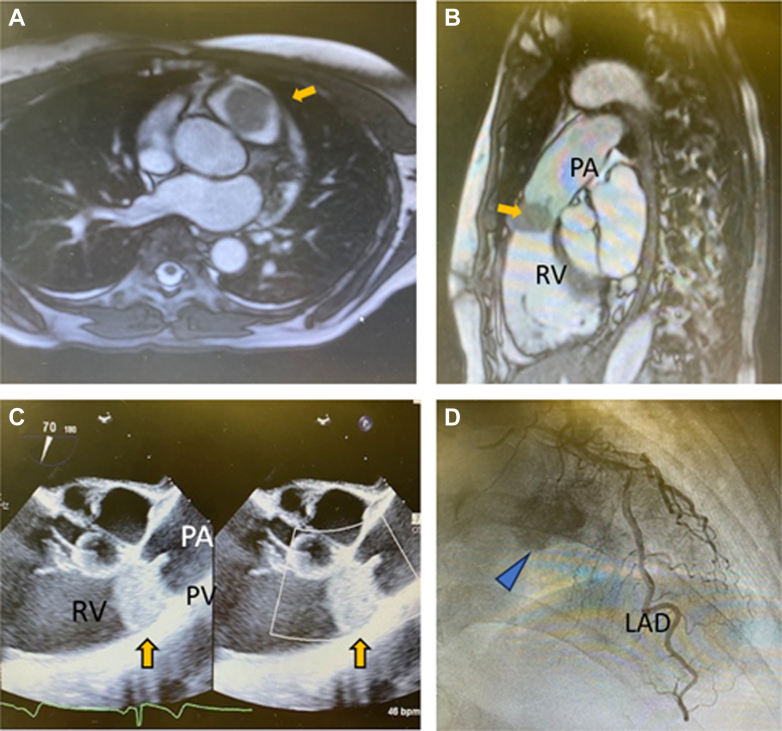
Preoperative Imaging (A) Cardiac magnetic resonance (axial slice) with mass (yellow arrow) in right ventricular outflow tract. (B) Sagittal image of cardiac magnetic resonance showing mass (yellow arrow) with close association of the pulmonary valve (PV). (C) Echocardiography demonstrating mass and involvement with PV with right ventricular outflow tract obstruction. (D) On left heart catheterization, the mass opacifies (blue arrow) with contrast agent injected into the left main coronary artery. LAD = left anterior descending coronary artery; PA = pulmonary artery; RV = right ventricle.
